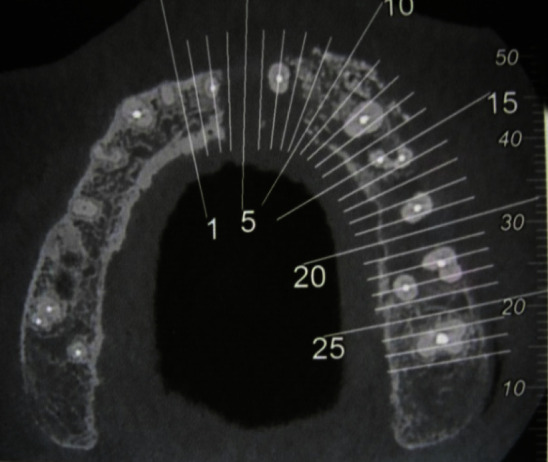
CBCT (Cone-Beam Computed Tomography) view: large radiolucent lesion between the teeth 11 - 27
